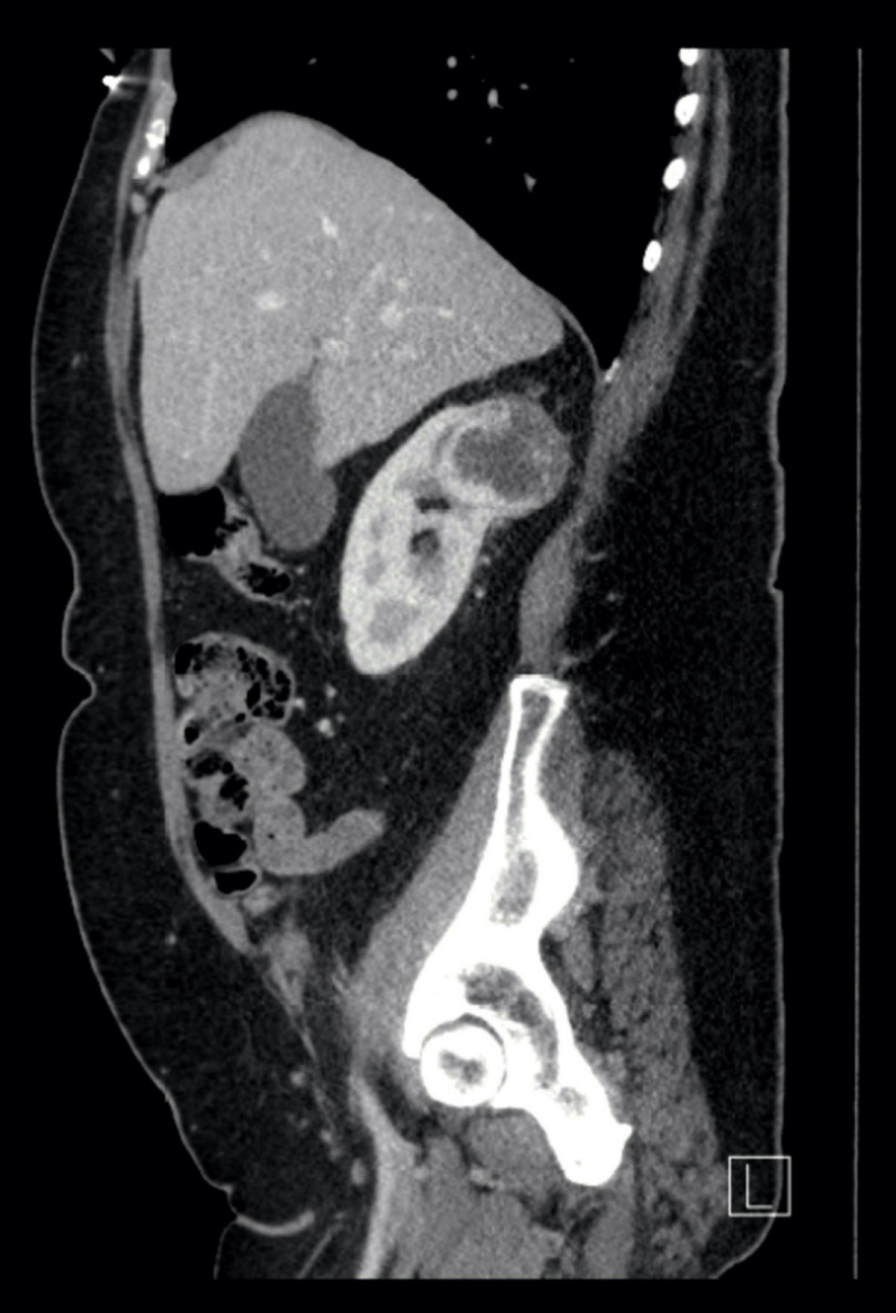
Sagittal CT view showing upper pole right renal tumour.
